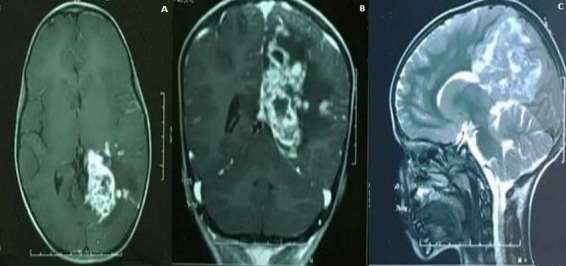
A) axial T1 weighted MR image showing left parieto-occipital necrotic nodules with high signal intensity and meningeal granulations; B) coronal T1 weighted MR image with gadolinium injection showing peripheral enhancement of the lesions; C) sagittal T2 weighted MR image showing nodules with low signal intensity and peripheral edema
